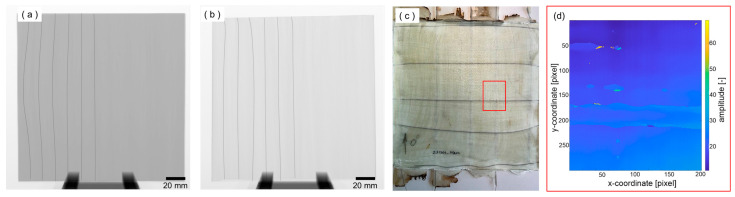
Computer tomography images of a sample with (a) additional aluminum cover layers on both sides and (b) without cover layers. Image of the sample and marked in red (c), where the ultrasonic measuring area is located. C-scan of the measuring area with runtime as color (d).
